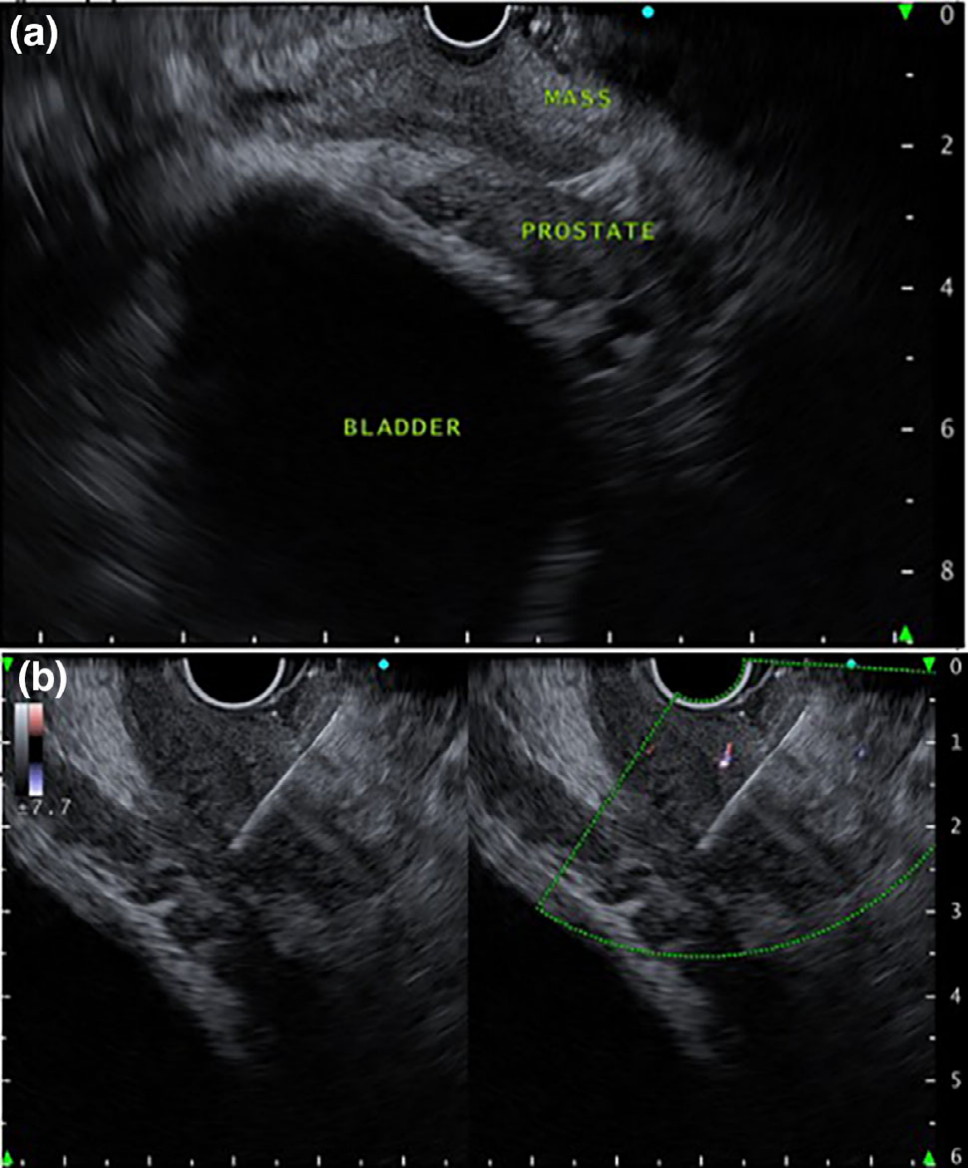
Findings of endoscopic ultrasound of the rectum. A submucosal hypoechoic mass lesion with the possibility of prostatic invasion was observed (a). Endoscopic ultrasound‐guided fine needle biopsy was performed from the lesion (b).
